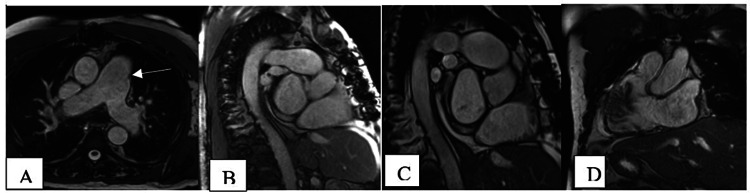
Cardiac MRI. A: Axial T2 images shows dilated main pulmonary artery with narrowing of main pulmonary artery atanastomotic site (White arrow). B and C: Short axis TRUFI images shows short segment narrowing of main pulmonary artery. D: TRUFI RVOT image shows dilated main pulmonary artery
